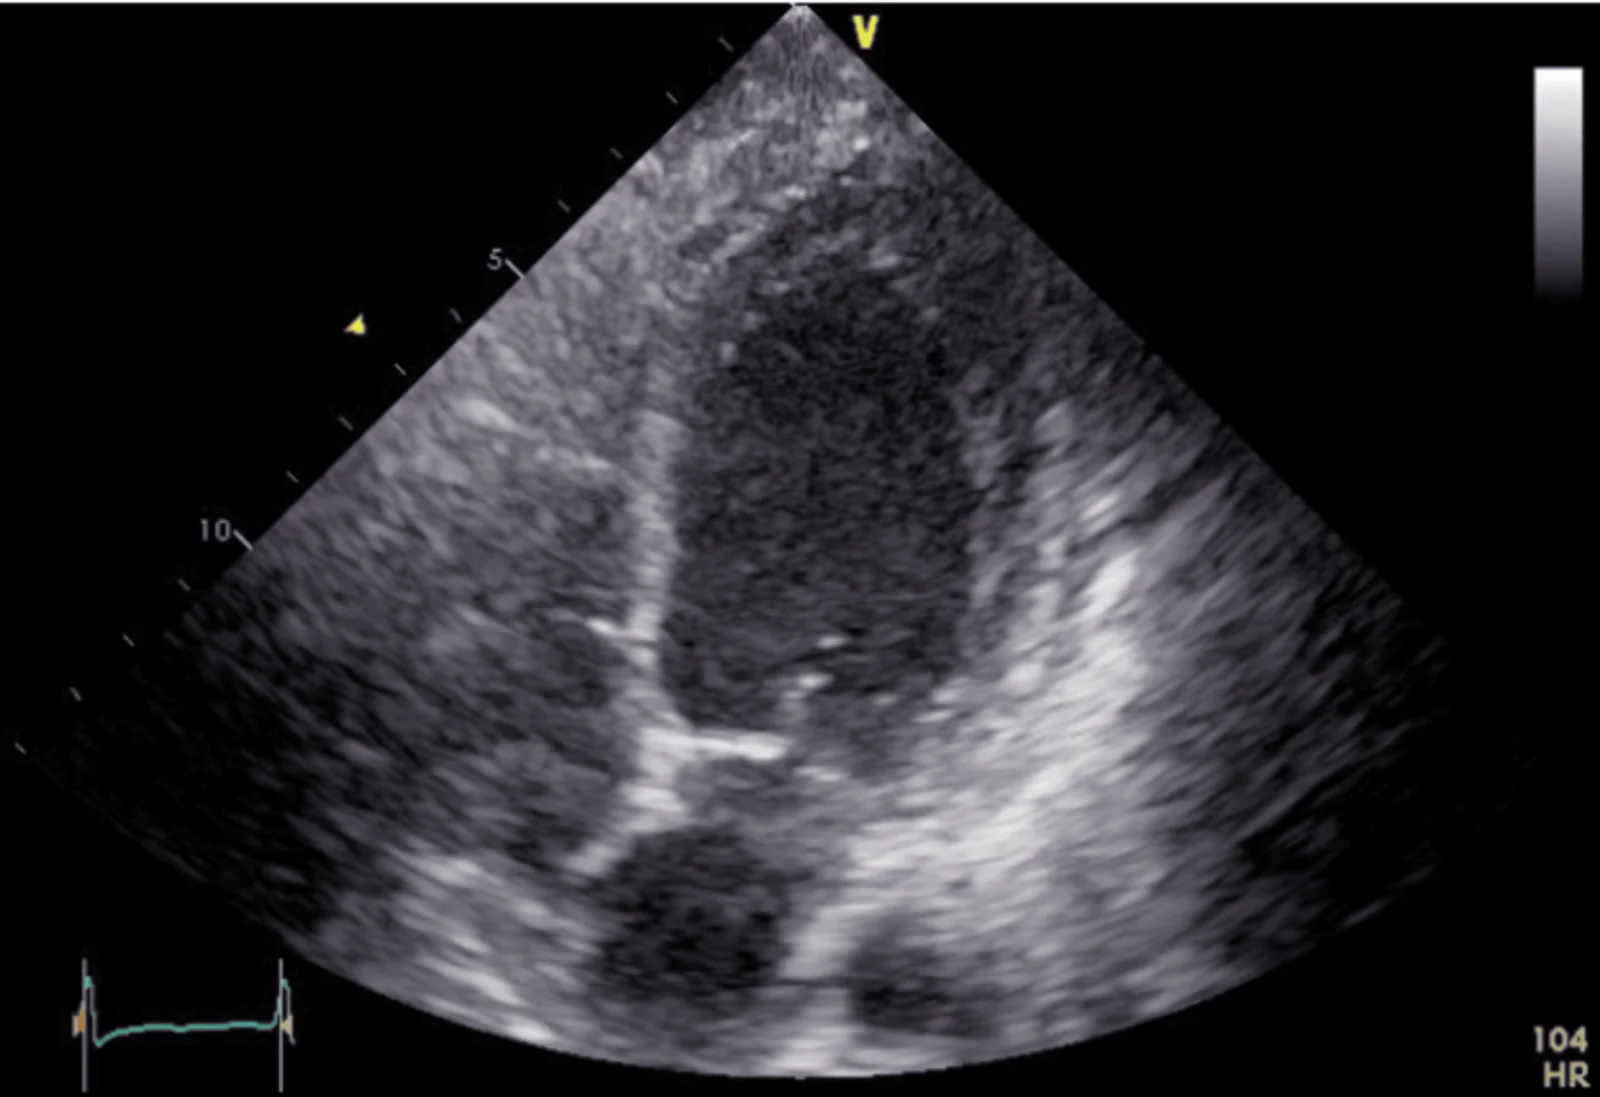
Transthoracic echocardiography on admission Transthoracic echocardiography demonstrated severe diffuse left ventricular systolic dysfunction with a left ventricular ejection fraction of 28%. No left ventricular thrombus was observed.
